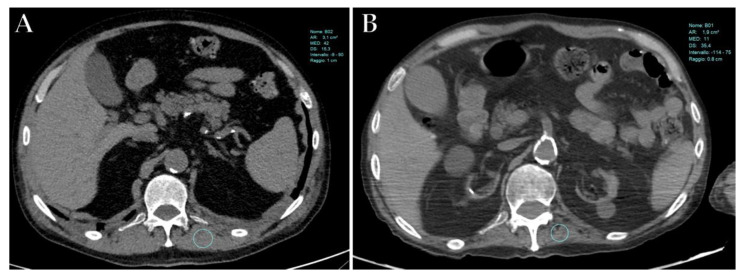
Axial unenhanced CT images of a patient with severe COVID-19 at the same level (T12), performed at the time of the ICU admission (A) and after a 3-month of ICU stay (B), demonstrates a reduction in muscle mass in the left paravertebral muscle (i.e., <30 HU) during hospitalization.
